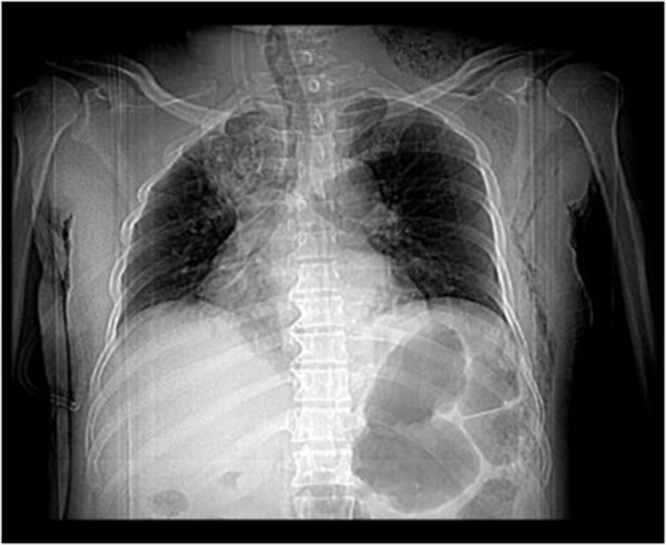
Preliminary chest X-ray showing a right superior mediastinal opacity with multiple left chest wall gas shadows.
